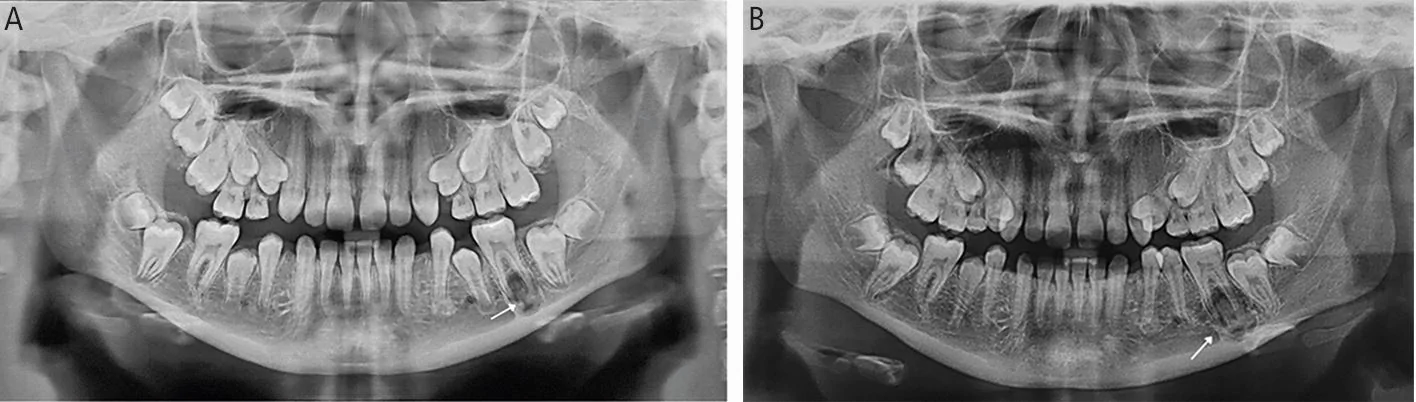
Panoramic radiographs of Case 2 at 13 years (A) and 15 years (B), demonstrating progression of the mixed-density lesion between the roots of tooth 36 (arrows), multiple unerupted permanent teeth, and prolonged retention of primary teeth
